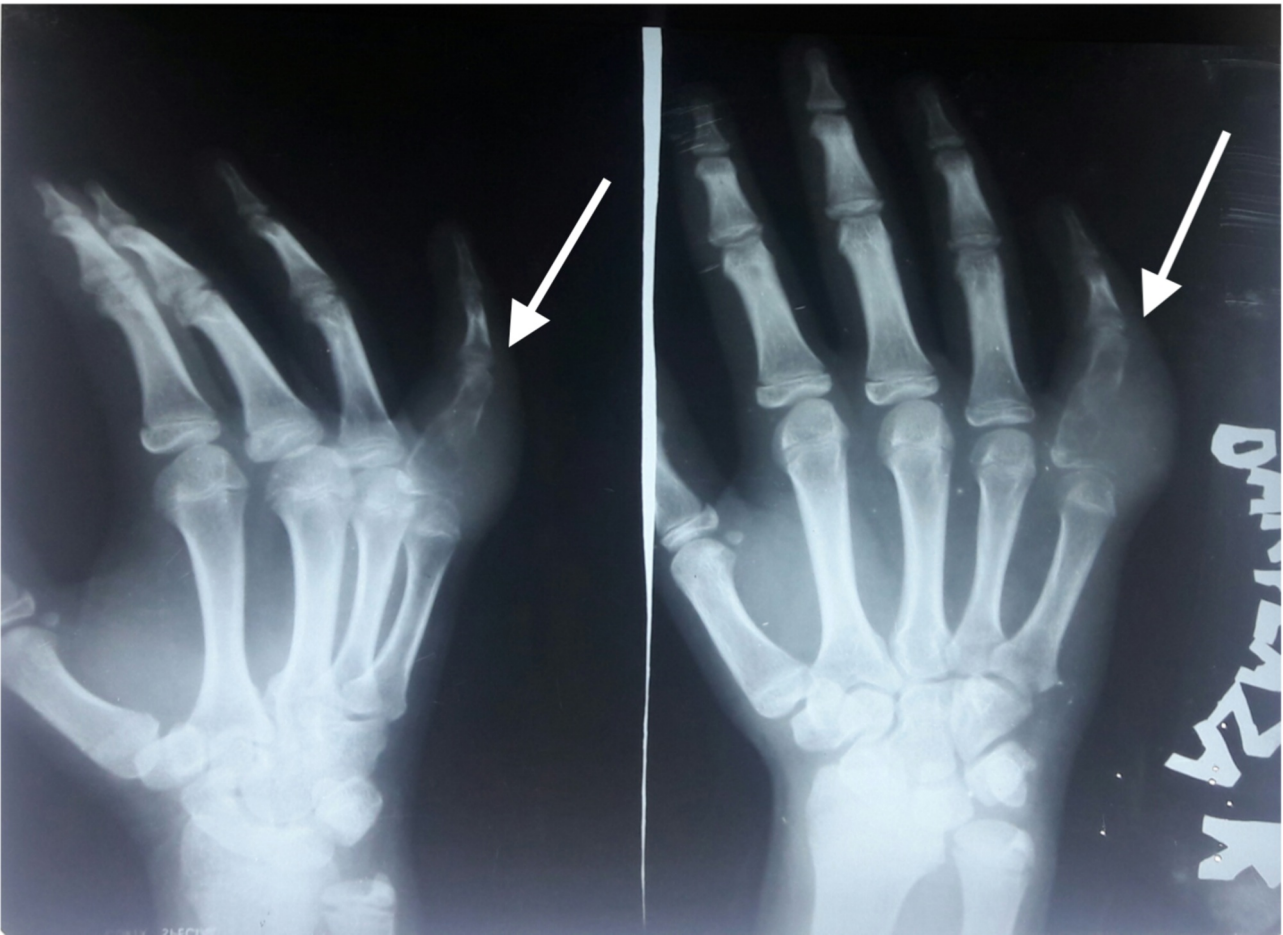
X-rays showing a malignant tumor originating from bone and infiltrating the soft tissue (white arrow).
